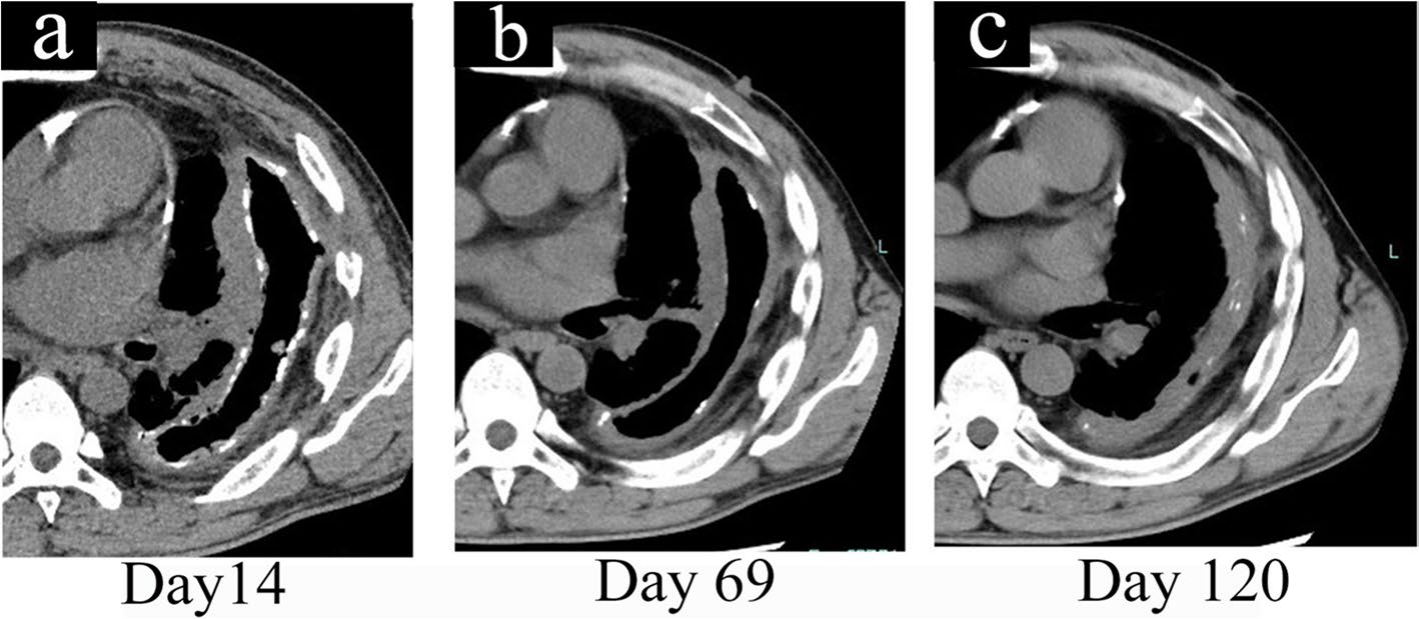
Postoperative CT findings of the second surgery (fenestration surgery). Postoperative CT showed that there was a reduction in the calcified pleura and empyema cavity with lung expansion by daily decortication for 77 days followed by NPWT for 43 days. a 14 days after the 2nd surgery. b 69 days after the 2nd surgery. c 120 days after the 2nd surgery
